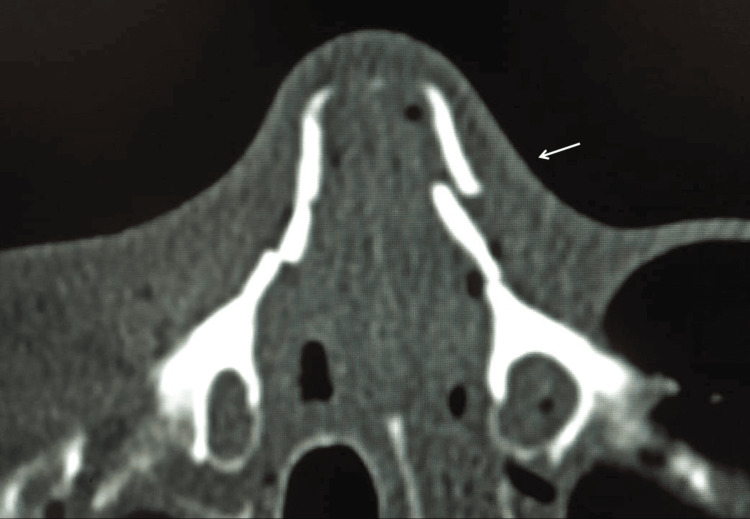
Axial computed tomography scan of a displaced nasal bone fracture The scan serves as the reference standard, clearly showing a distinct fracture of the nasal bone (arrow) with cortical disruption and displacement.
